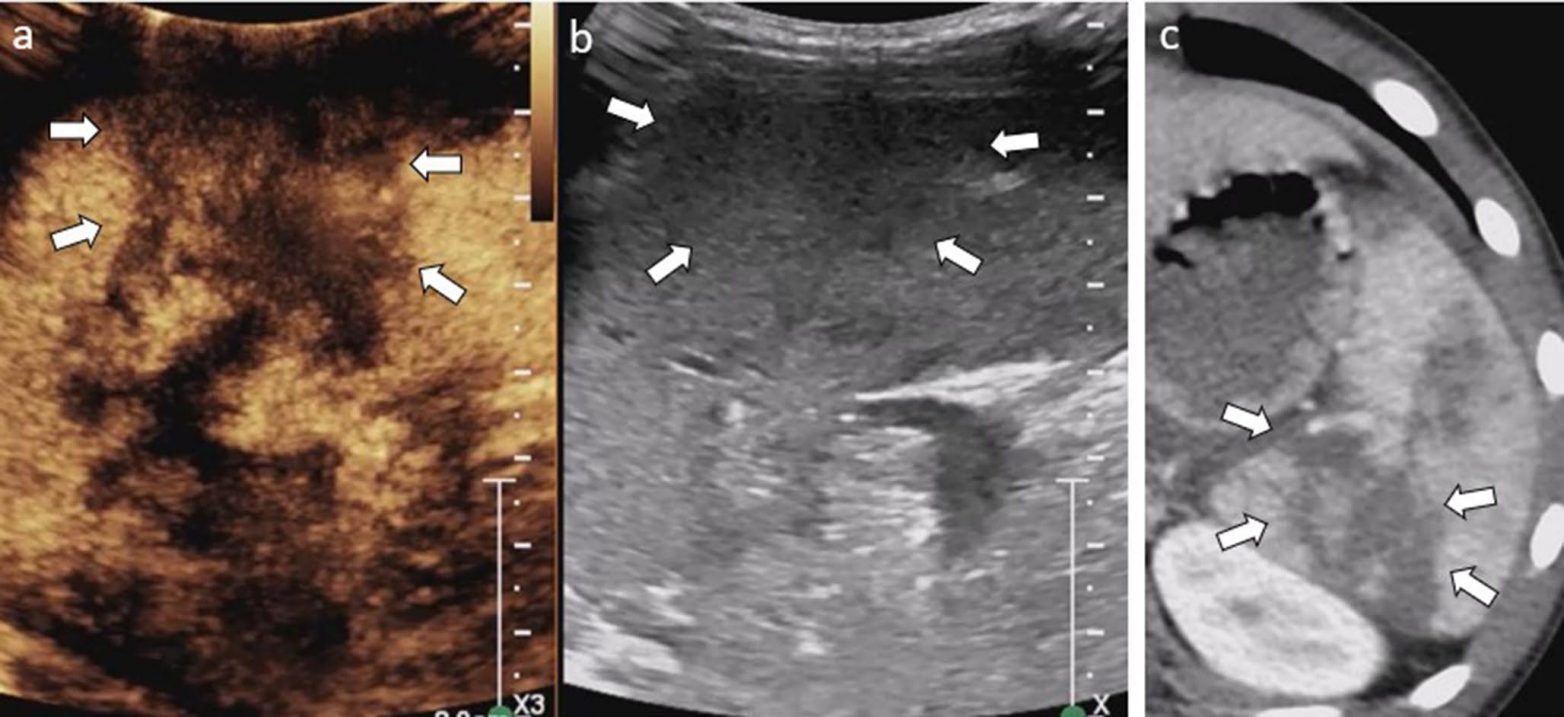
Splenic laceration in a pediatric patient. Sagittal contrast-enhanced ultrasound (CEUS) image of the spleen with a dual display of contrast (a) and gray-scale (b) modes. CEUS image reveals irregular hypoenhancing lacerations extending from the hilum to the capsule (arrows); however, the gray-scale shows only subtle hypoechogenicity in the subcapsular parenchyma (arrows). (c) Axial intravenous contrast-enhanced CT confirms a splenic laceration (arrows)
